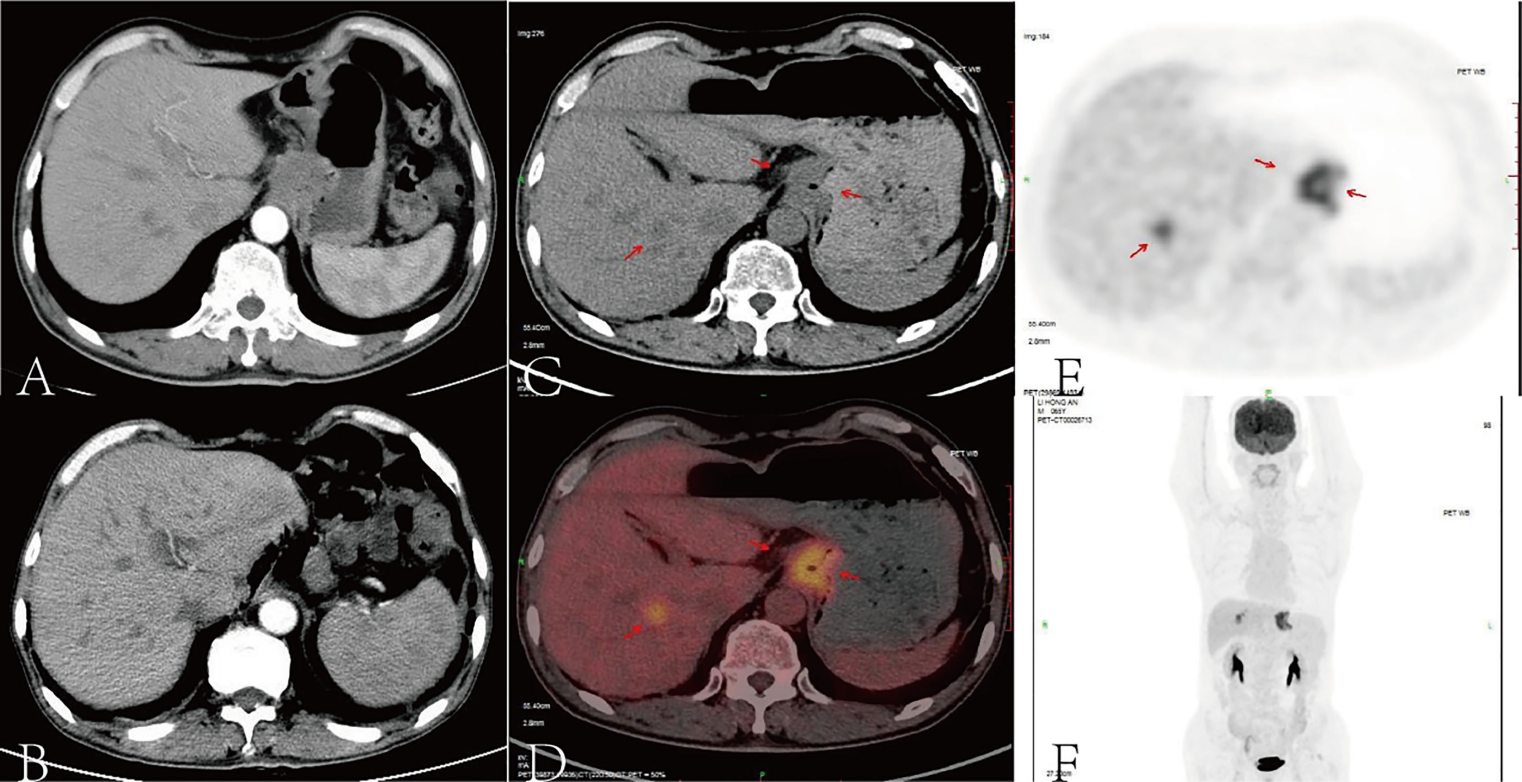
According to an abdominal contrast-enhanced computed tomography (CT) scan, there were a malignant gastric mass and seven low-density shadows in the liver recognized as liver metastases (A). After eight cycles of SOX, contrast-enhanced CT scan showed no recurrence of the primary tumor, but liver metastases did not show any obvious changes (B). After surgery and chemotherapy for gastric cancer, the stomach was absent, and the lower esophagus and jejunum were anastomosed. A high-density metal anastomotic line shadow (indicated by the arrow) can be seen at the anastomotic stoma, and a small patch of slightly increased radiation uptake shadow can be seen locally. A small nodular low-density shadow with a size of about 0.5 cm can be seen in the right lobe of the liver (S8 segment). The density and radioactivity distribution in other parts of the liver were not abnormal. A small nodule-calcified shadow can be seen under the capsule of the right lobe of the liver (S6 segment). According to the positron emission tomography (PET) scan, the red arrows pointed out the tumor foci (C–E), the image (F) showed the image of coronal plane.
